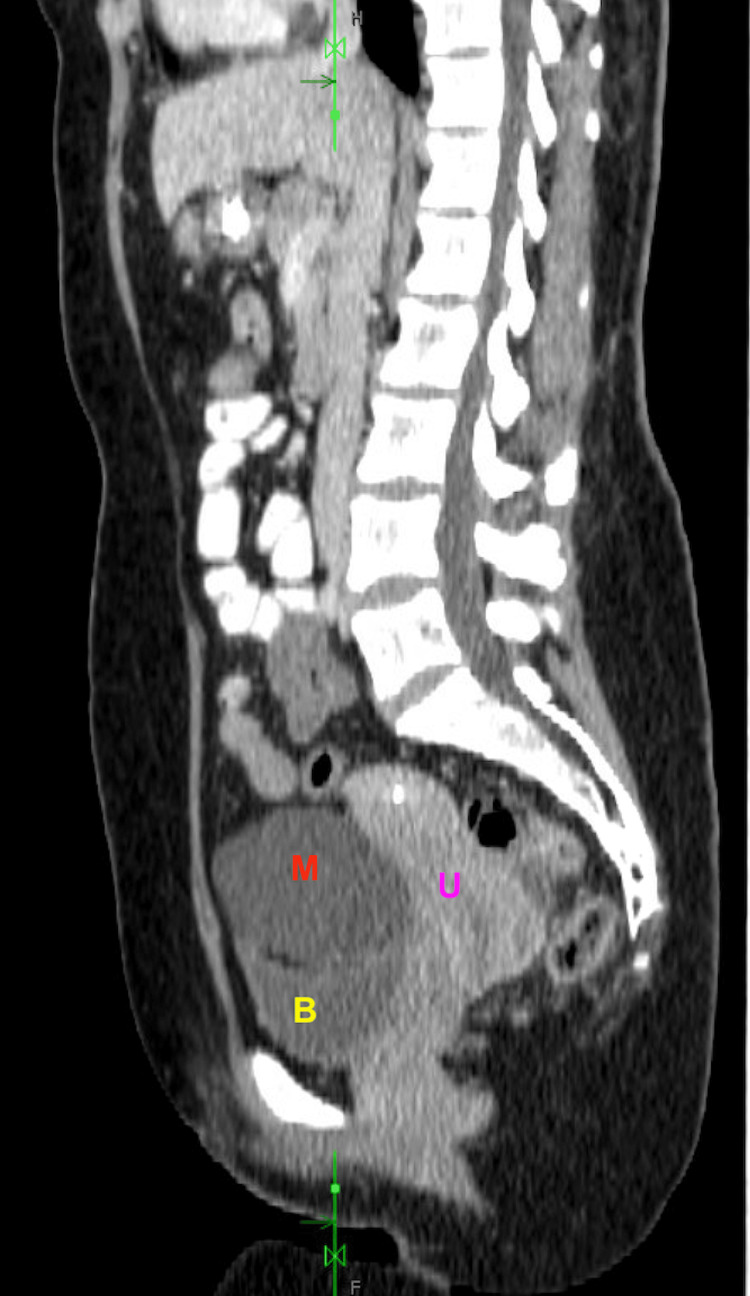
CT abdomen and pelvis demonstrating mesothelial cyst Labels: M represents the mesothelial cyst, B denotes the bladder, and U indicates the uterus
